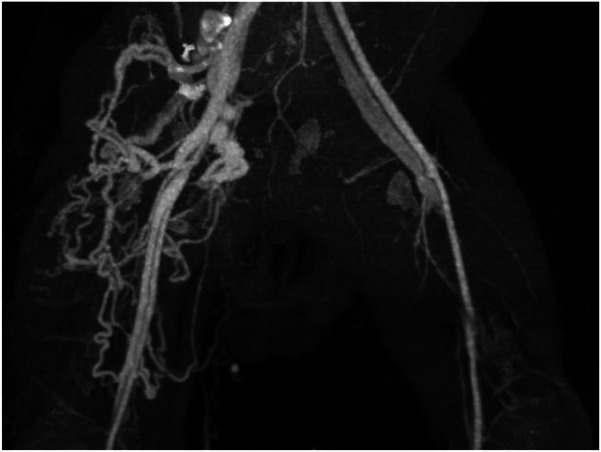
CT angiogram demonstrating bilateral arteriovenous fistulas. The right-sided fistula is supplied by a markedly dilated and tortuous femoral artery and its branches, which drain into a large, tangled venous plexus. This plexus consists of dilated and tortuous femoral and great saphenous veins. In contrast, the left-sided fistula is characterized by moderate dilation of the femoral artery and vein.
